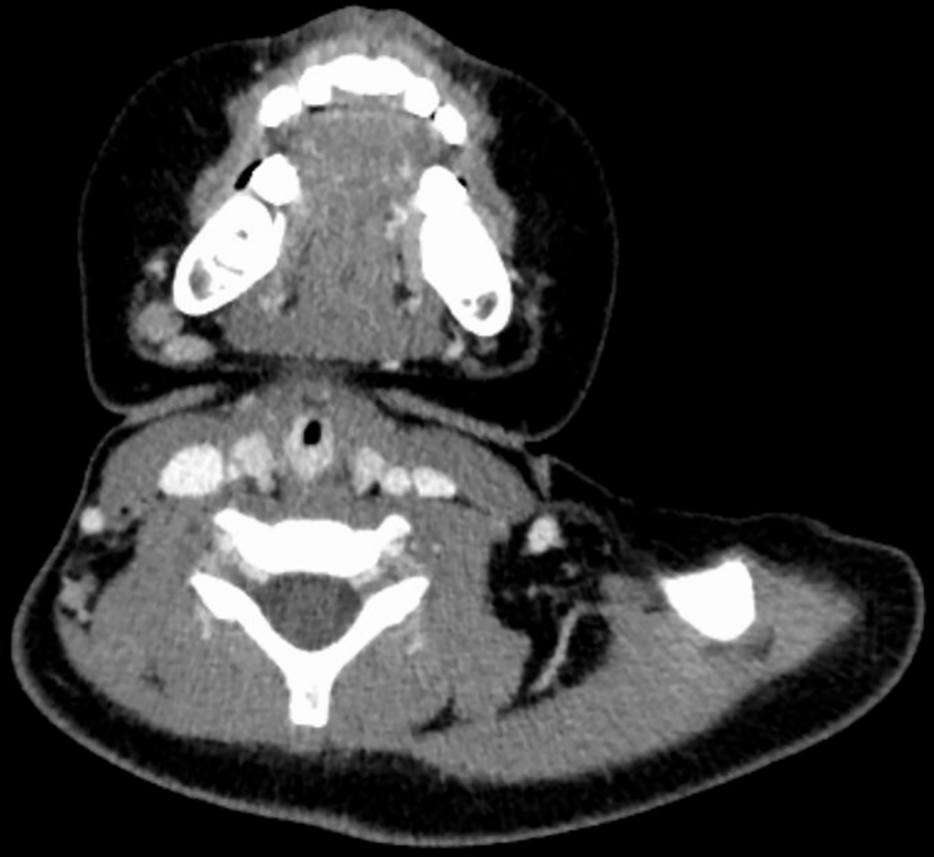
CT image of No. 8 patient before embolization treatment, with marked enhancement of hemangioma and airway stenosis.
